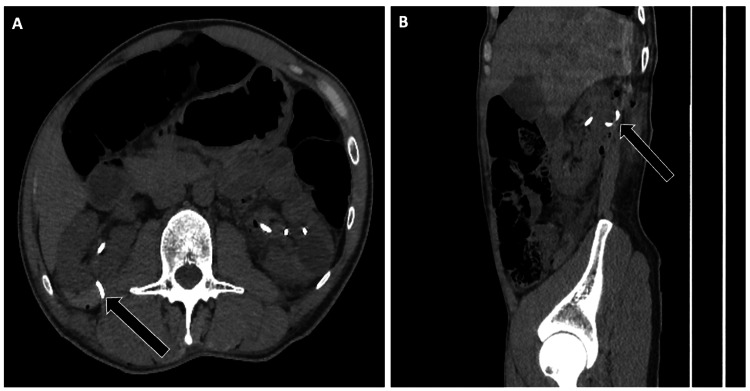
Proximal Malposition of a Ureteral Stent With Renal Cortex Perforation Non-contrast CT of the abdomen and pelvis demonstrating malposition of a right ureteral stent. (A) Axial image shows the proximal stent tip extending beyond the posterior renal cortex (arrow). (B) Sagittal reconstruction confirms extrarenal location of the stent tip with an associated perirenal hematoma (arrow). This complication highlights the importance of verifying stent position relative to the renal collecting system to avoid parenchymal injury.
